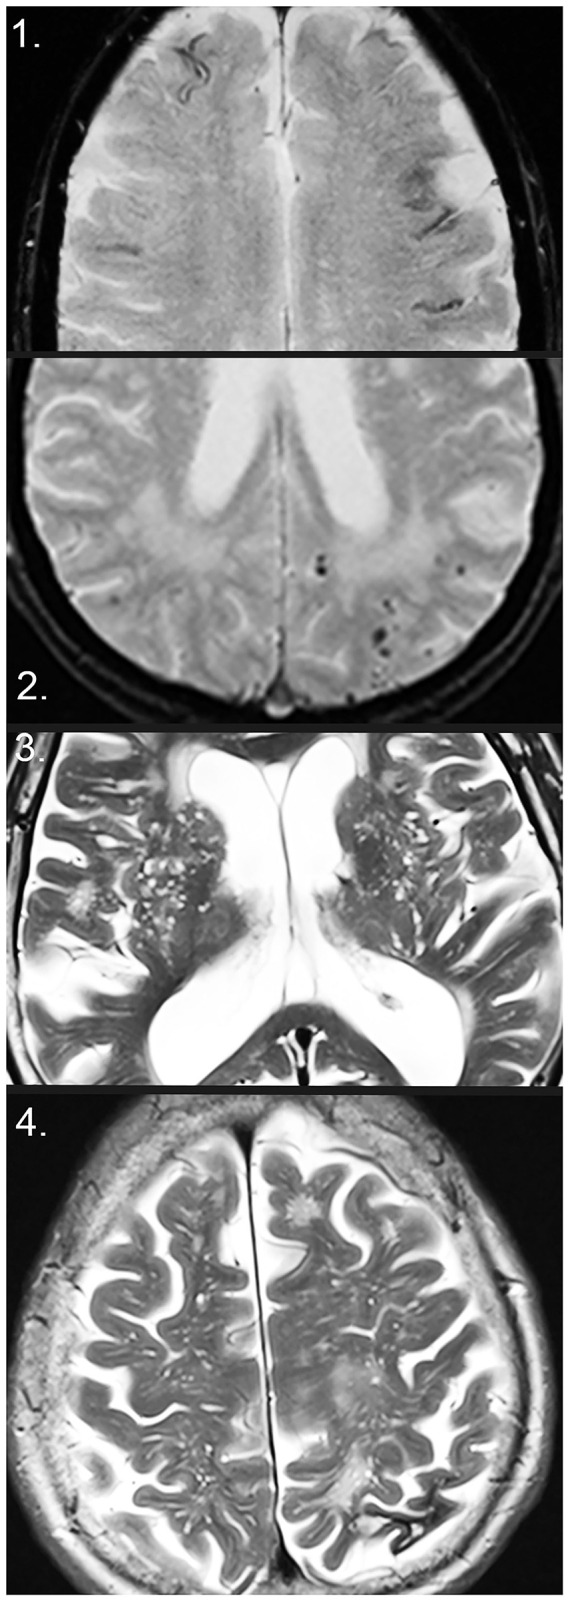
Markers of small vessel disease on MRI. (1) Disseminated superficial siderosis on T2*. (2) Cerebral microbleeds (CMBs), as well as white matter hyperintensities on T2*. (3) Enlarged perivascular spaces (EPVS) in the basal ganglia on T2. (4) EPVS in the centrum semiovale on T2 and intracerebral macrohemorrhage with local atrophy in the left parietal lobe.
